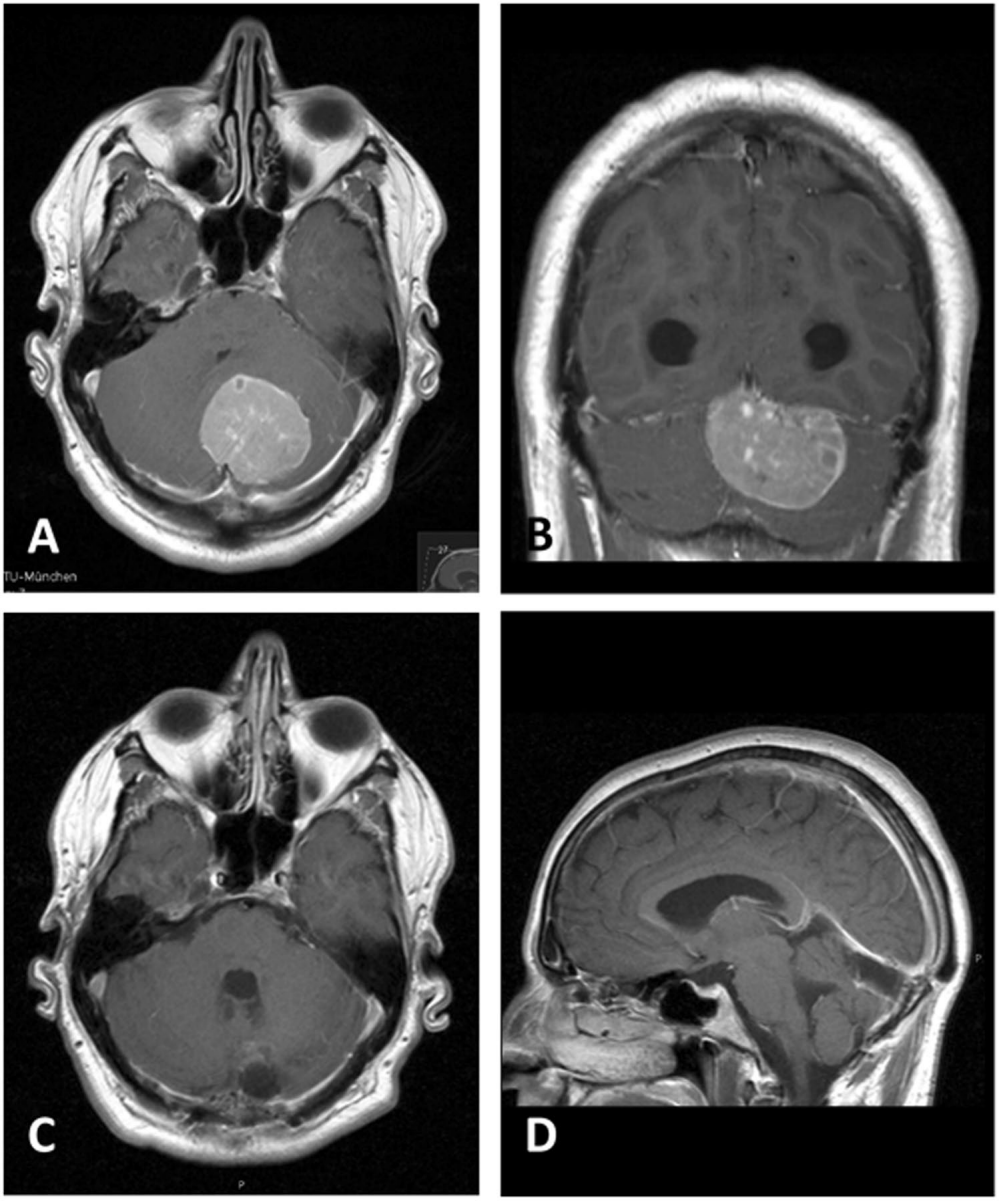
Medial* infratentorial meningioma in a 43-year-old male who presented with accentuated headaches, nausea and vomiting. Preoperative contrast-enhanced T1 MRI in axial (A) and coronal (B) reconstructions. A transient gait ataxia was noted postoperatively. Imaging after resection (S2) via a median suboccipital craniotomy (C,D).
